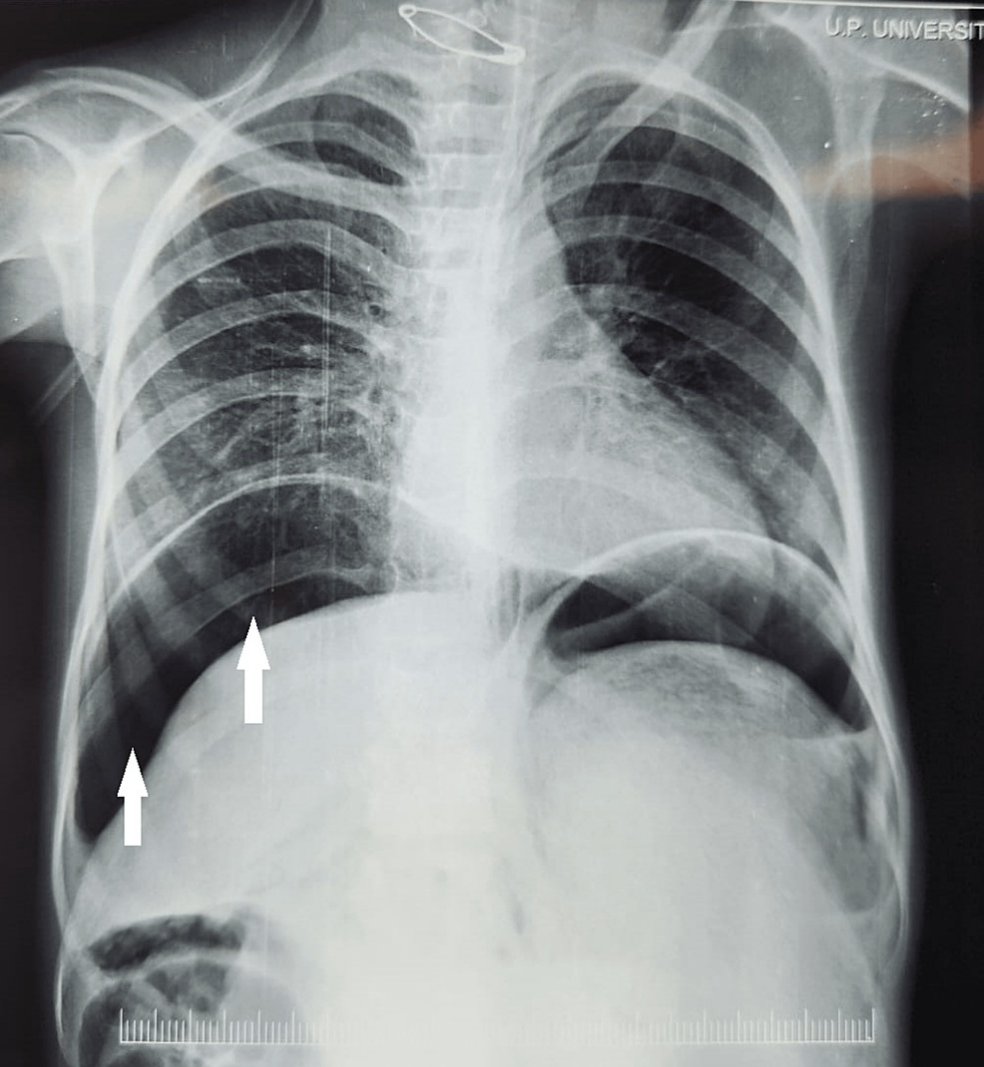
Gas under diaphragm seen in erect chest X-ray (marked by white arrows).
